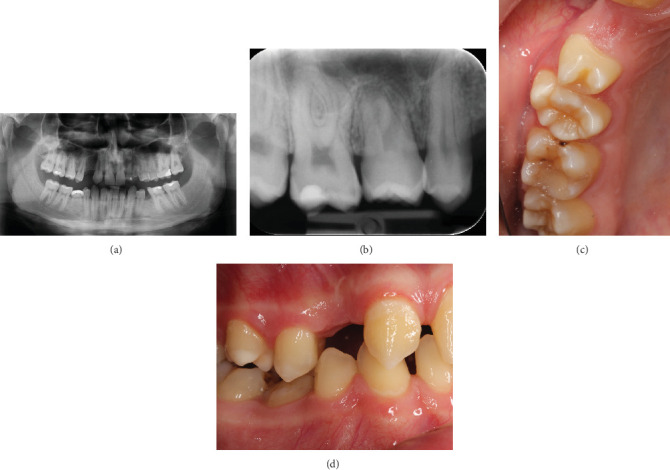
At the 1-year follow-up visit. From (a) the orthopantomogram and (b) the periapical radiograph, we see that the root formation has continued, pulpal obliteration is evident, and there is no sign of pathology. (c) Occlusal view. (d) Side view.
